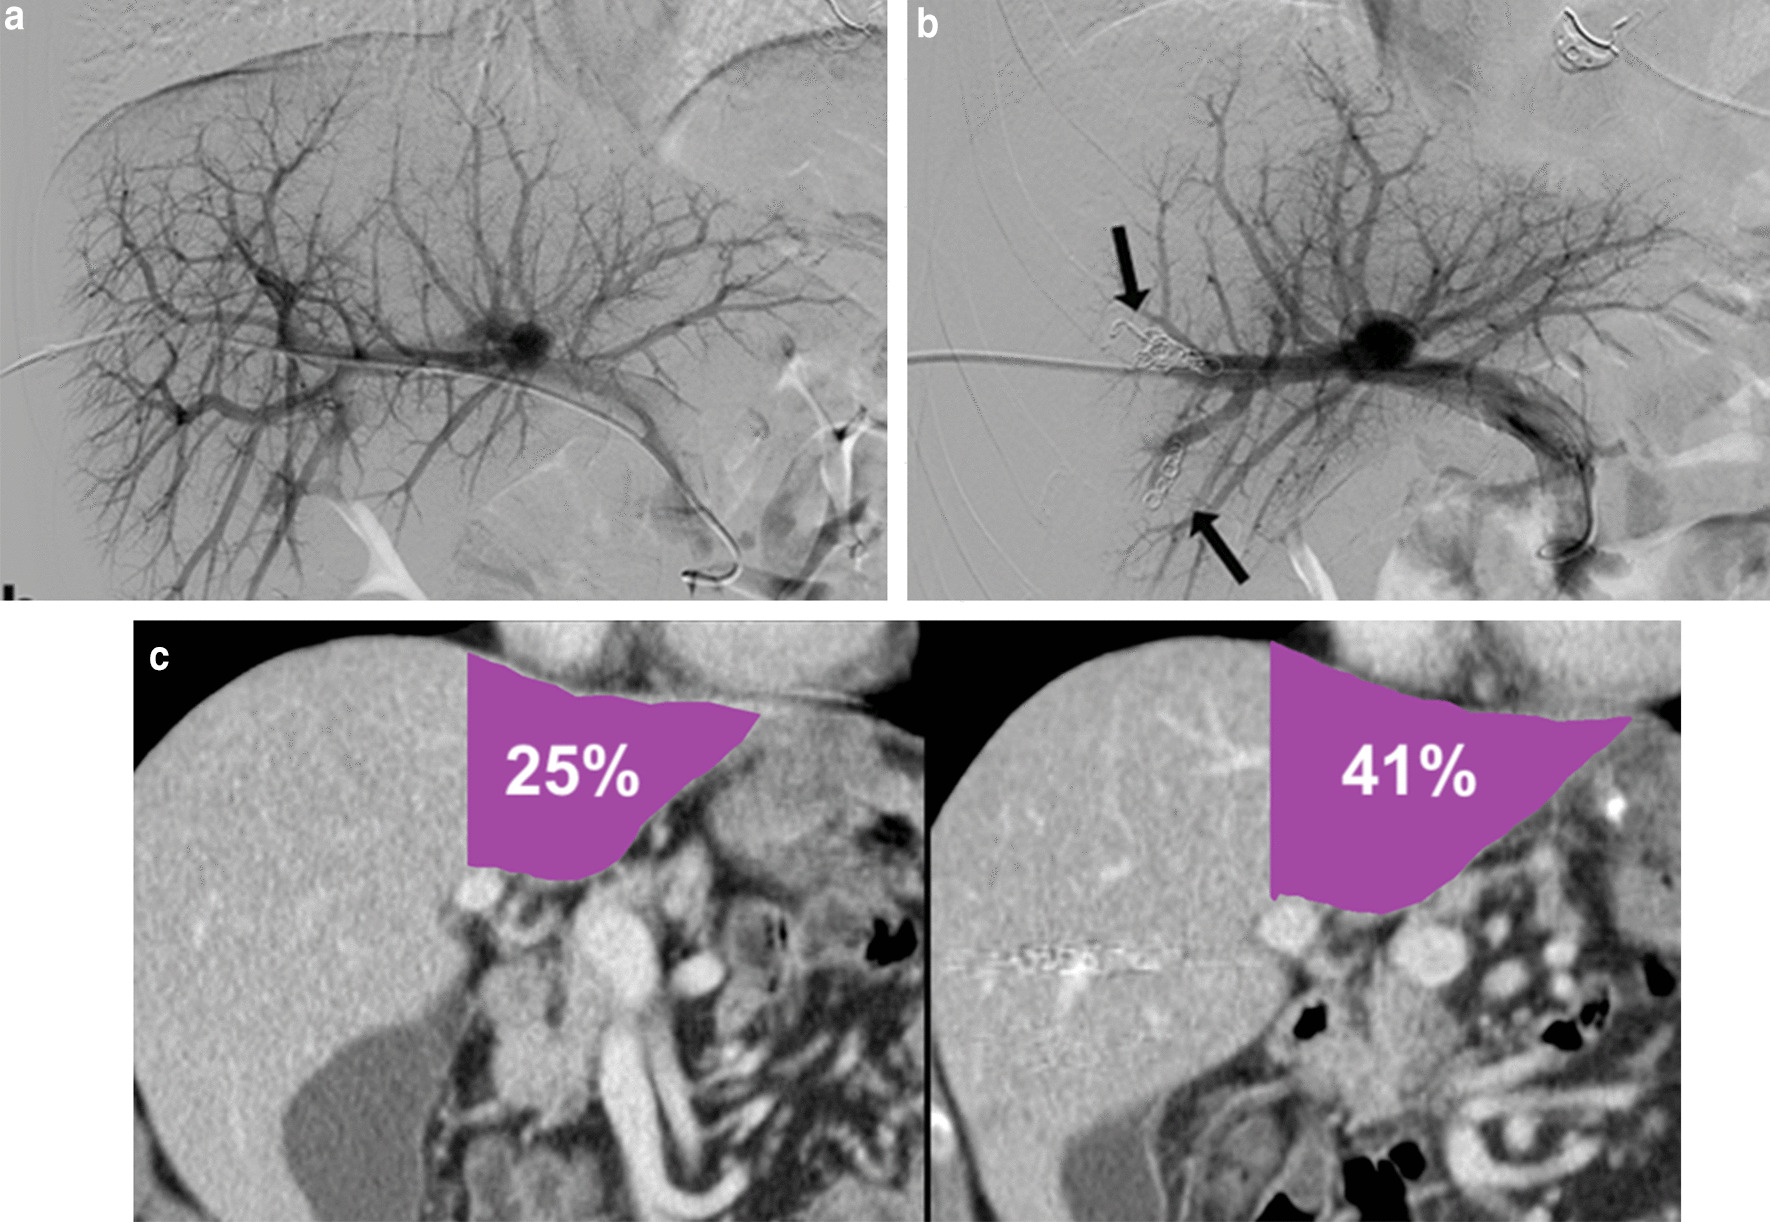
A Portography acquired immediately before portal vein embolization shows normal portal vein anatomy. B Control portography immediately after portal vein embolization with microspheres and coils shows successful occlusion of the right portal vein branches. C Computed tomography images before portal vein embolization and 4 weeks after showing significant hypertrophy of the left liver lobe (future liver remnant increased from 25 to 41%)
